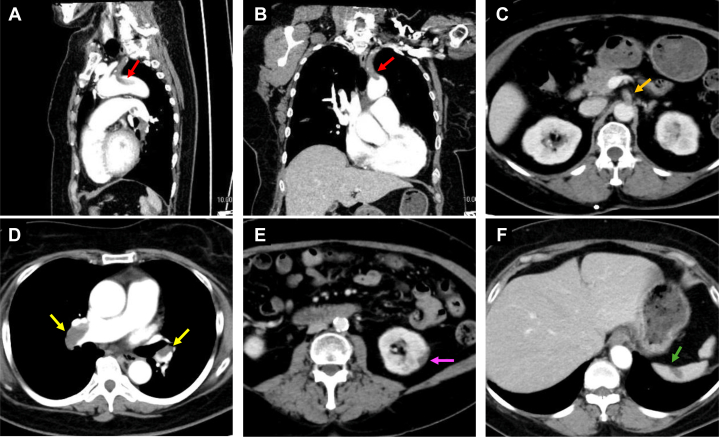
Contrast-Enhanced Computed Tomography Findings at the Time of Stroke Center Consultation (A and B) Thrombi were observed extending from the left subclavian artery to the aortic arch, with protruding thrombi in the aortic arch (red arrows). (C to F) Multiple thrombi were present in the celiac artery (C, orange arrow) and pulmonary arteries (D, yellow arrow), with evidence of renal infarction (E, purple arrow) and splenic infarction (F, green arrow).
